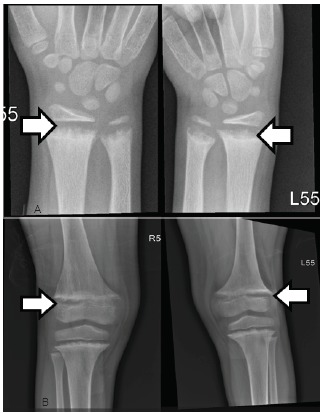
Radiograph of bilateral wrists (A) and knees (B) of an eight-year-old boy with new-onset seizure. Arrows denote areas of poor bone mineralization.
